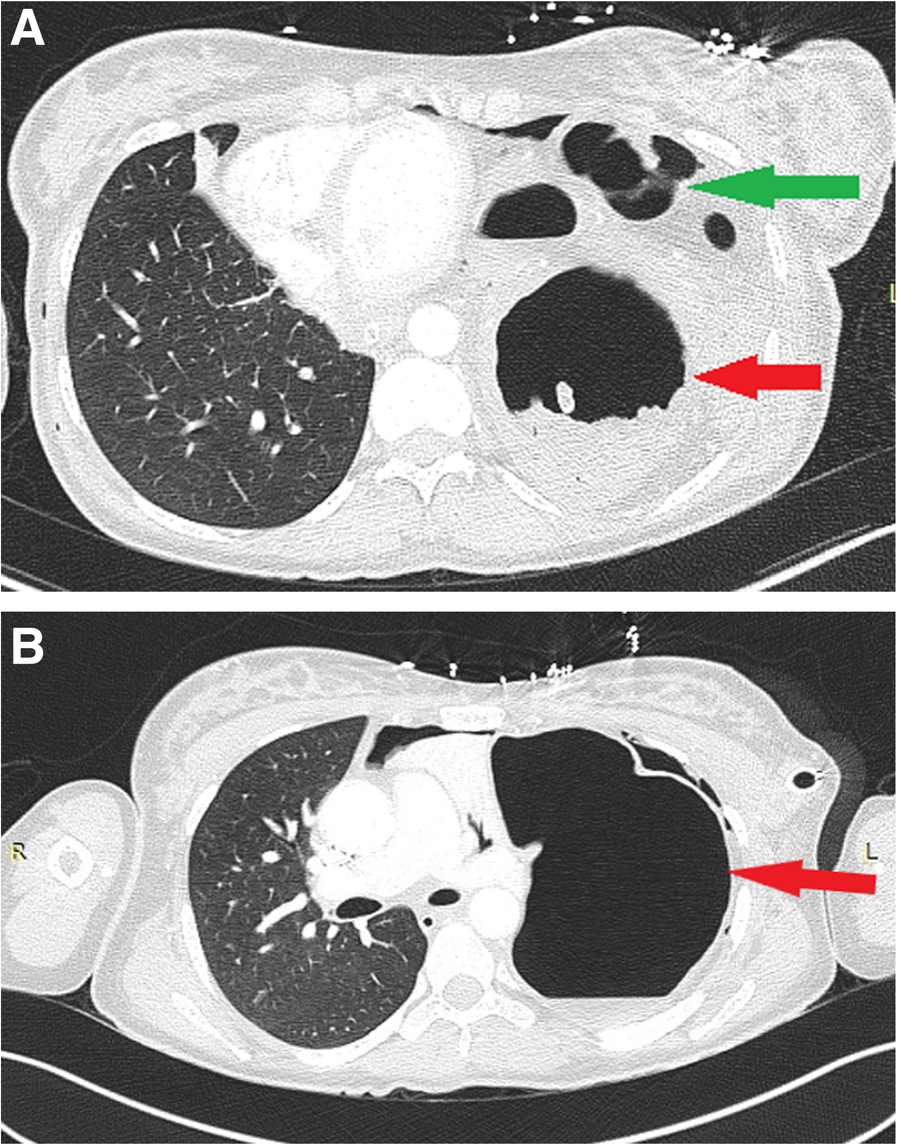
a and b Chest and Abdomen computed tomography scan (CT) showing the whole stomach (Red arrow), part of the small bowel and the colon (Green arrow) herniating into the left hemithorax
